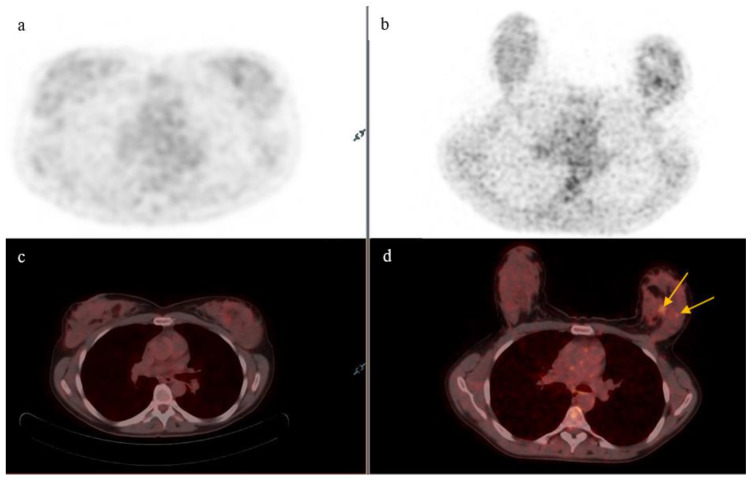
Axial PET and fused PET/CT images of the breast. Two small lesions (yellow arrows) are visualized within the left breast in prone acquisition (b,d), corresponding to multi-focal disease. Supine acquisition (a,c) showing single focal uptake in the left breast.
